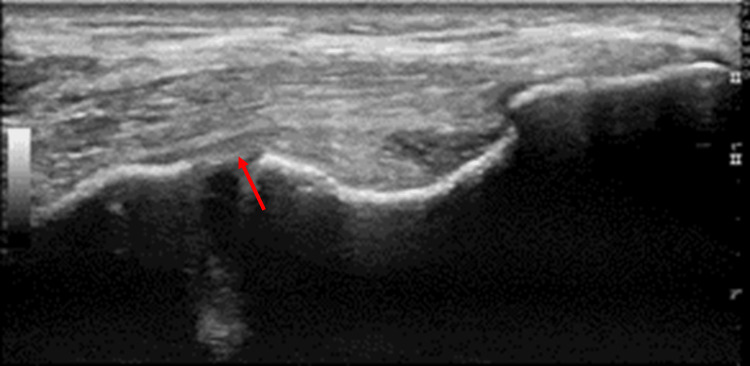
Post-treatment 12-week ultrasound. Demonstrates improved ligamentous echotexture and a healthy-appearing ulnar collateral ligament (arrow).
